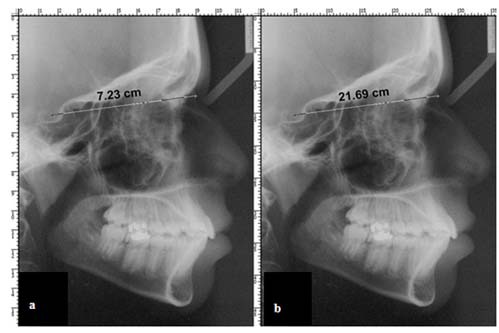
a: A cephalograph of 1416 × 1888 pixels2 with a resolution of 300 DPI. The to-be-printed size of this example radiograph (indicated by the Photoshop® rulers around the image) is 11.99 × 15.99 cm2. The SN measurement is reported 7.23 cm by the Photoshop® measuring tool. b: The same 1416 × 1888-pixel image, however, with a spatial resolution edited to 100 DPI. Its printing size is obviously different (35.97 × 47.96 cm2). The SN measurement is calculated as 21.69 cm using Photoshop®.
